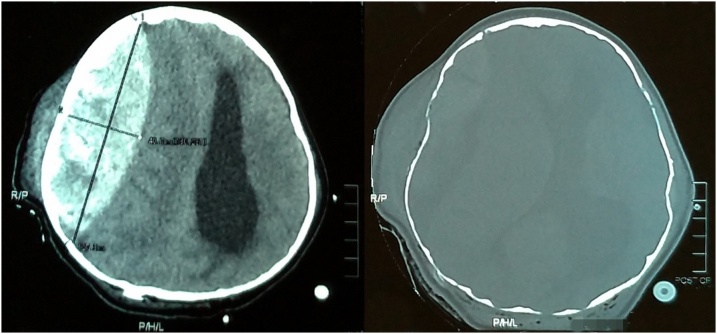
An emergent CT head evaluation shows bilateral depressed skull fracture and a massive epidural hematoma with 40 mm thickness causing 10 mm midline shift to the left.
